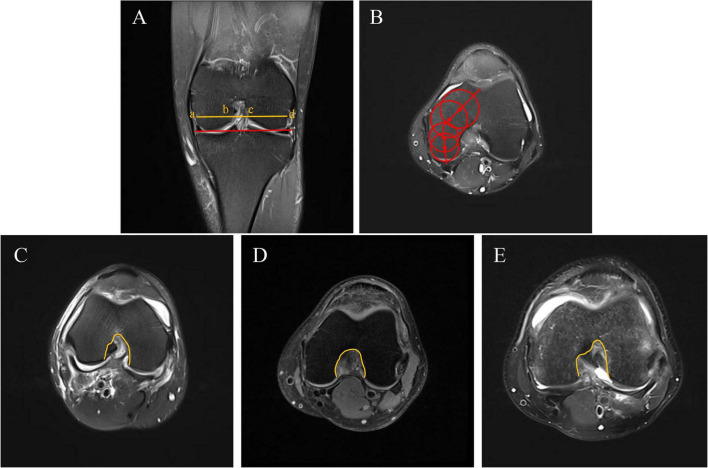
Measurement of osseous morphologic parameters on coronal and axial MRI. (A) The red line connects the distal ends of the medial and lateral femoral condyles. The yellow line is drawn at the level of the popliteal groove and parallel to the red line. Points *a* and *d* are defined at the medial and lateral distal condylar margins on the red line, and points *b* and *c* are defined at the medial and lateral borders of the intercondylar notch on the yellow line. The NWI was calculated as bc/ad, and the LFCWI as cd/ad. (B) The MFCA was defined as the angle formed by the two red lines. (C–E) Representative examples of intercondylar notch morphology: A-shaped (C), U-shaped (D), and W-shaped (E). NWI, notch width index; LFCWI, lateral femoral condyle width index; MFCA, medial femoral condyle angle.
